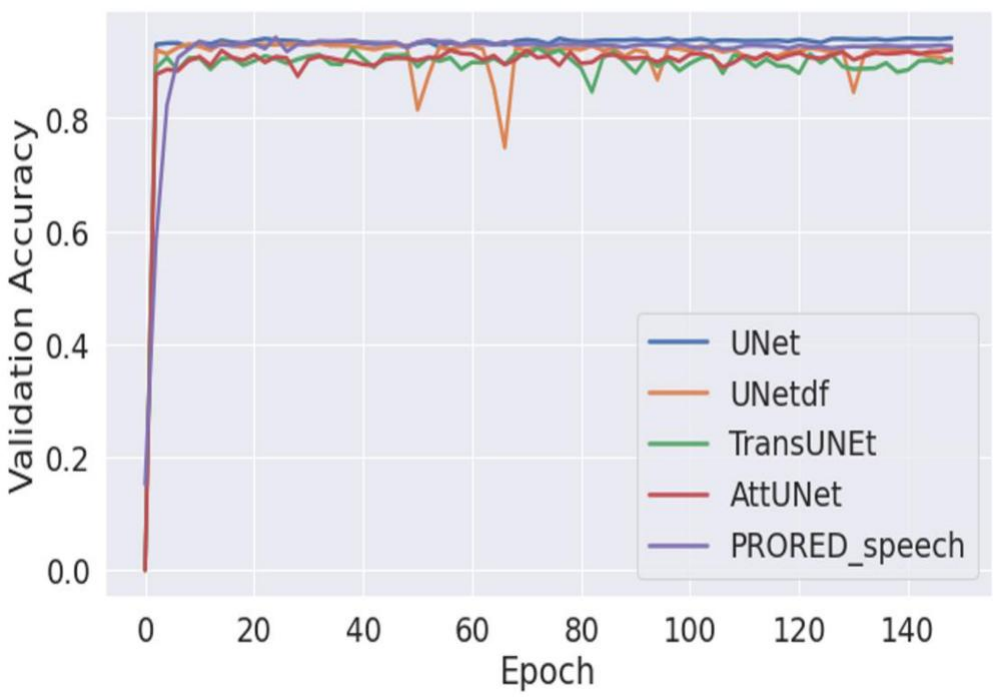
Validation accuracy plots for the baseline and proposed models from one of the folds on speech MRI data.
